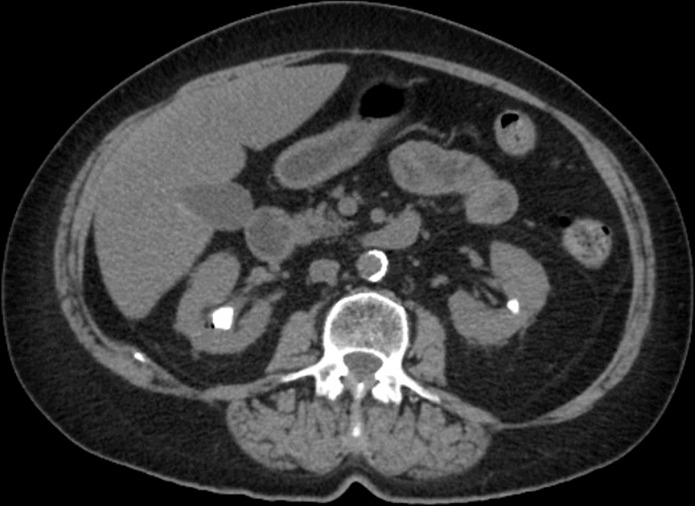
Abdominal and pelvic CT. The cut showed a 2.8 cm staghorn stone in the upper pole of the right kidney and a 8 mm non-obstructing stone in the interpolar region of the left kidney.
